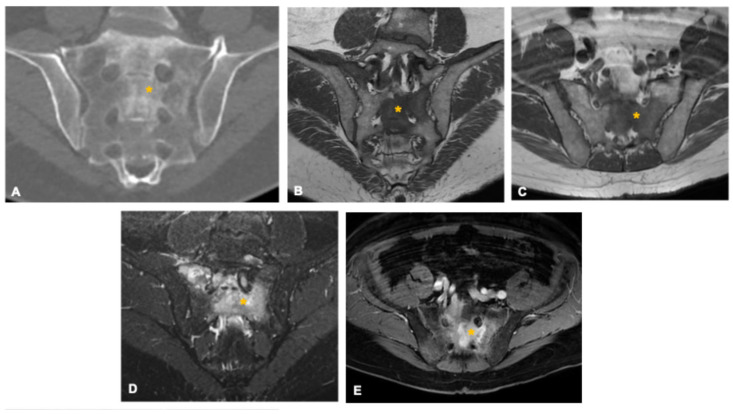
Primary bone lymphoma in a 71-year-old man. Coronal CT image (A) demonstrates a predominantly sclerotic, poorly defined sacral lesion (asterisk). Coronal (B) and axial (C) T1-weighted images, as well as coronal STIR (D), reveal a sacral lesion (asterisk) with low-to-intermediate signal intensity on T1 and predominantly high signal on T2, with poorly demarcated margins. On the post-contrast sequence (E) heterogeneous enhancement is observed, including a central focus of intense enhancement (asterisk), an important consideration when selecting a biopsy site to increase diagnostic yield, as inconclusive biopsy results are not uncommon in this type of neoplasm.
